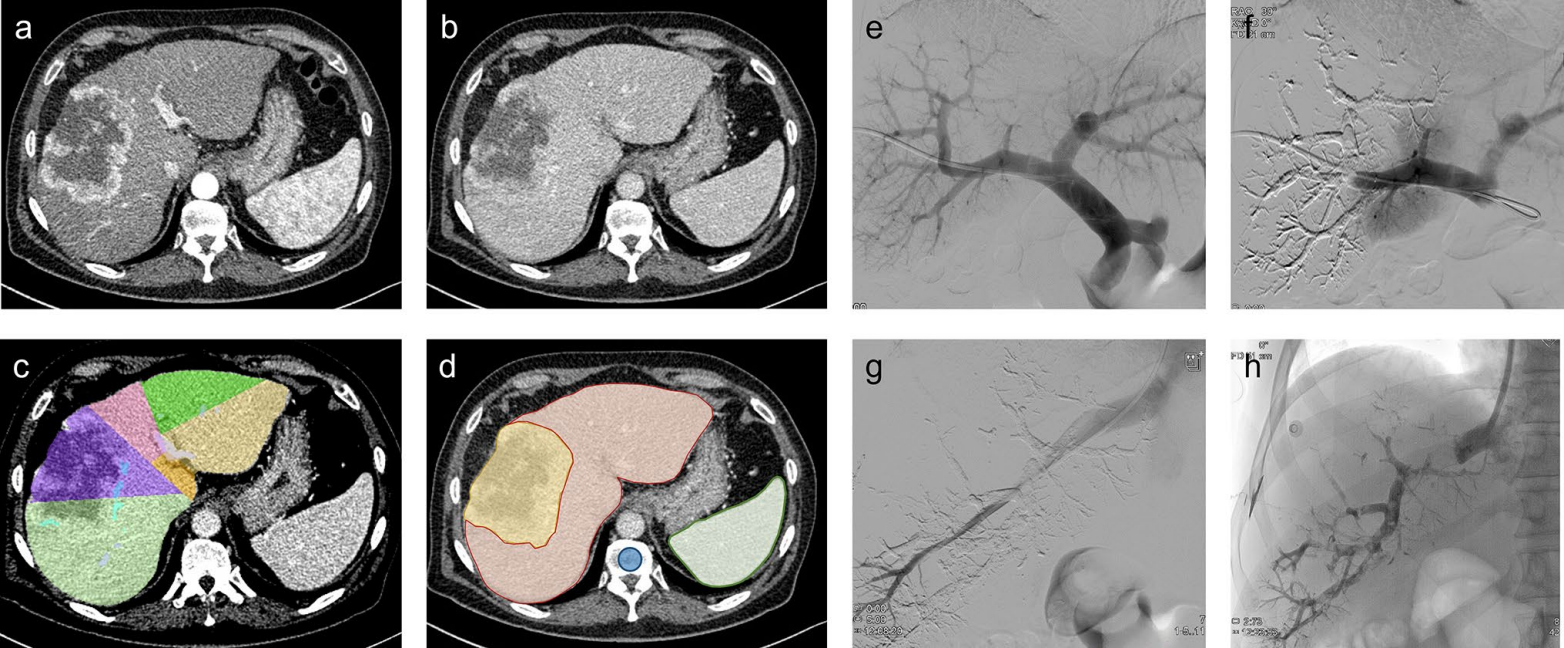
Representative CT imaging of a cholangiocarcinoma in the a arterial and b portal venous phases. c CT volumetry of the liver was performed to calculate FRLV and sFRLV. d Three-dimensional segmentation of the liver (without the tumor) and the spleen as well as ROI placement in the L1 vertebra for radiomics analysis was performed by two experienced radiologists in the portal venous phase of baseline CT. e–h Representative peri-interventional fluoroscopic images of a portal and hepatic vein embolization. *CT* Computed tomography, *FRLV* Future remnant liver volume, *sFRLV* Standardized future remnant liver volume, *ROI* Region of interest
